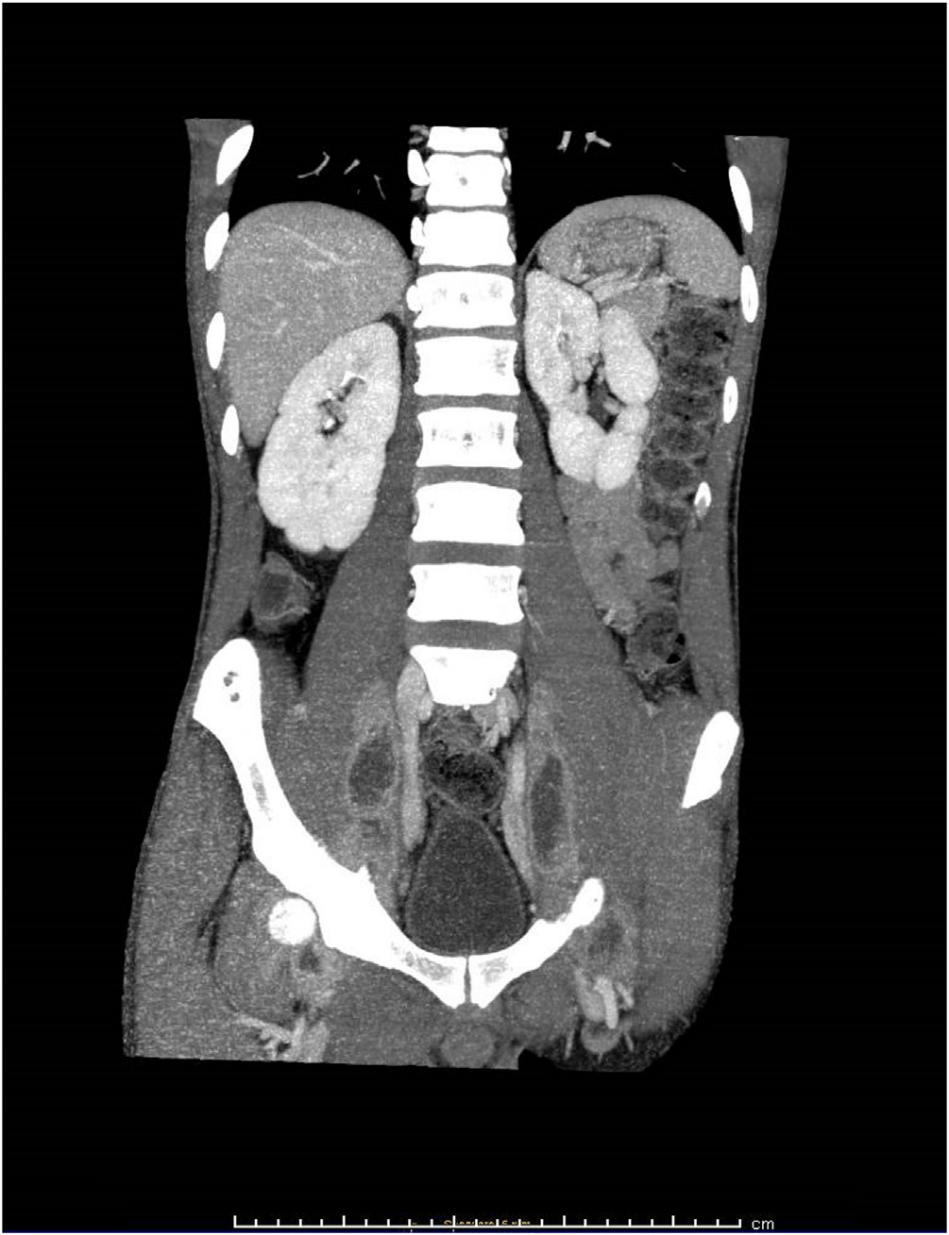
CT-scan coronal view: the abscesses were deeply in both the psoas muscles.
